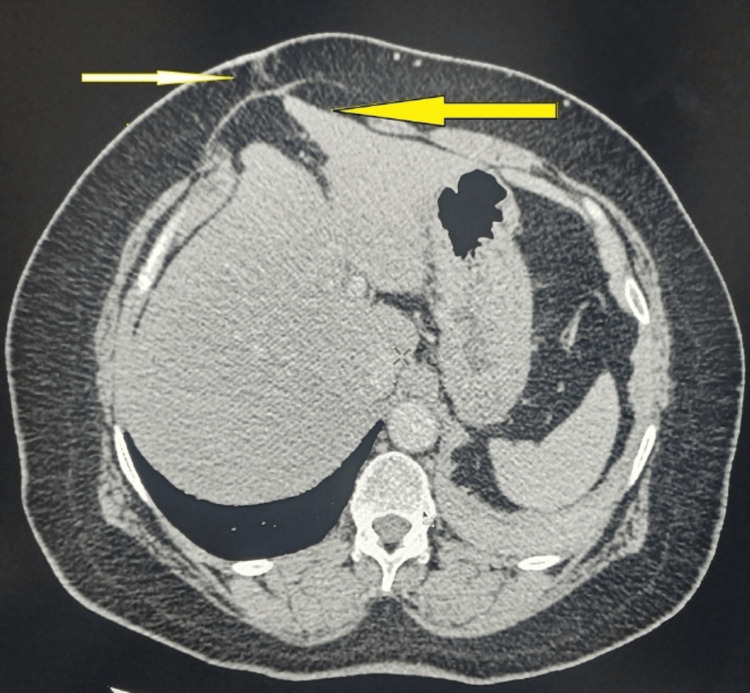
Axial section of CT scan abdomen showing surgical scar with hernial sac in it (white arrow), and herniation of liver at junction of right and left lobe in hernial sac through surgical scar (yellow arrow)
